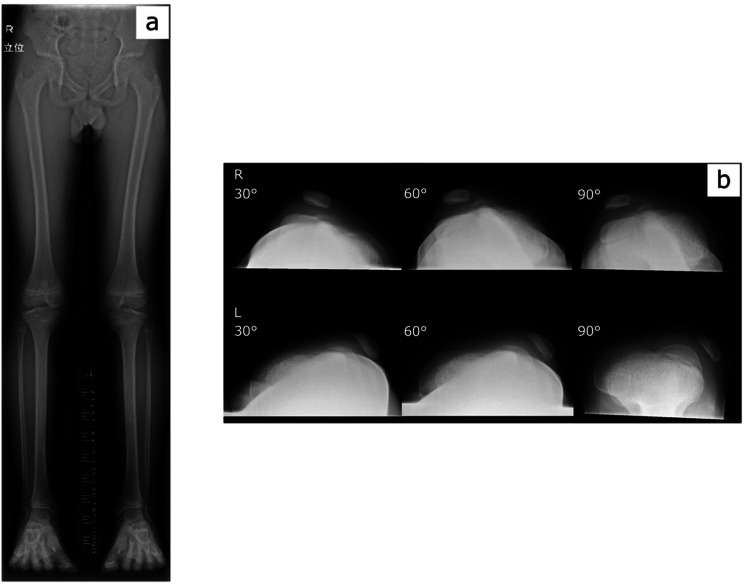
Standing radiograph and skyline images of the patella at 11 years of age Full-length radiographs of the standing lower limb (a) and skyline images of the patella (b) obtained before surgery on the left knee at 11 years of age.
